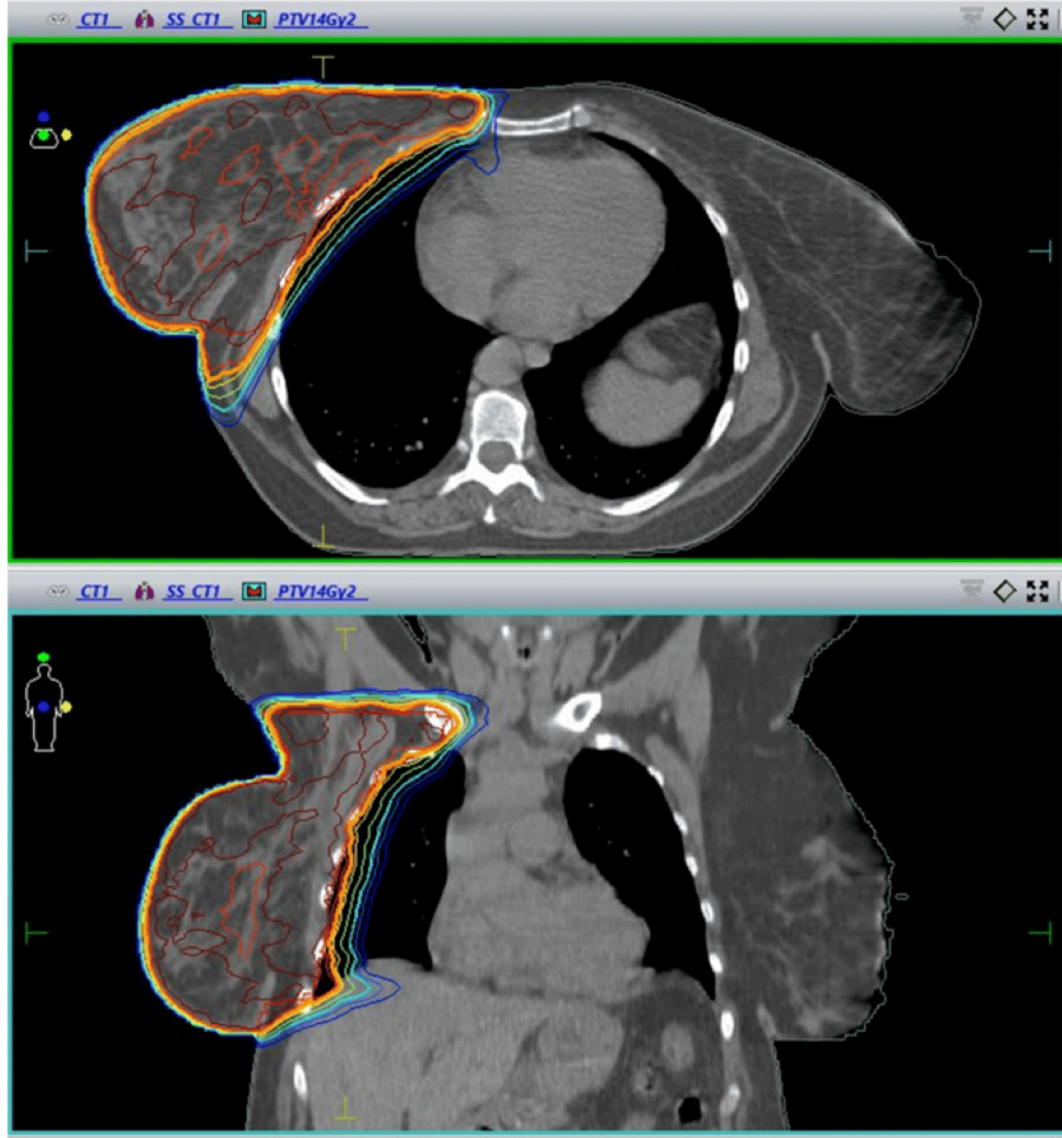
CT scan showing tumor formation.
